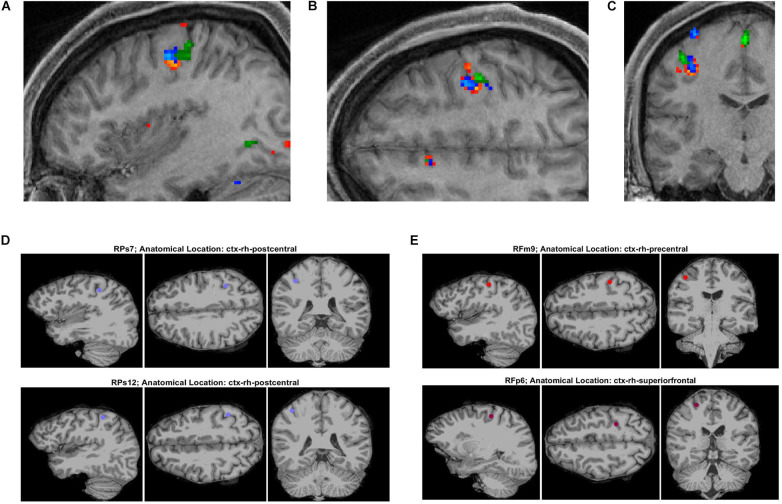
Functional MRI and post-surgery CT images for participant P3 related to finger tasks. (A–C) Functional MRI images in orthogonal planes (sagittal, transverse, and coronal) obtained during button press task for digits 1 (orange-red), 2 (blue), and 3 (green). (D,E) Post-surgical CT images of SEEG electrode sites found to have a repeatability metric (MCC) value greater than 0.6 (for time range of 0–4.4s with respect to cue onset) for flexion of digits 1 and 3.
